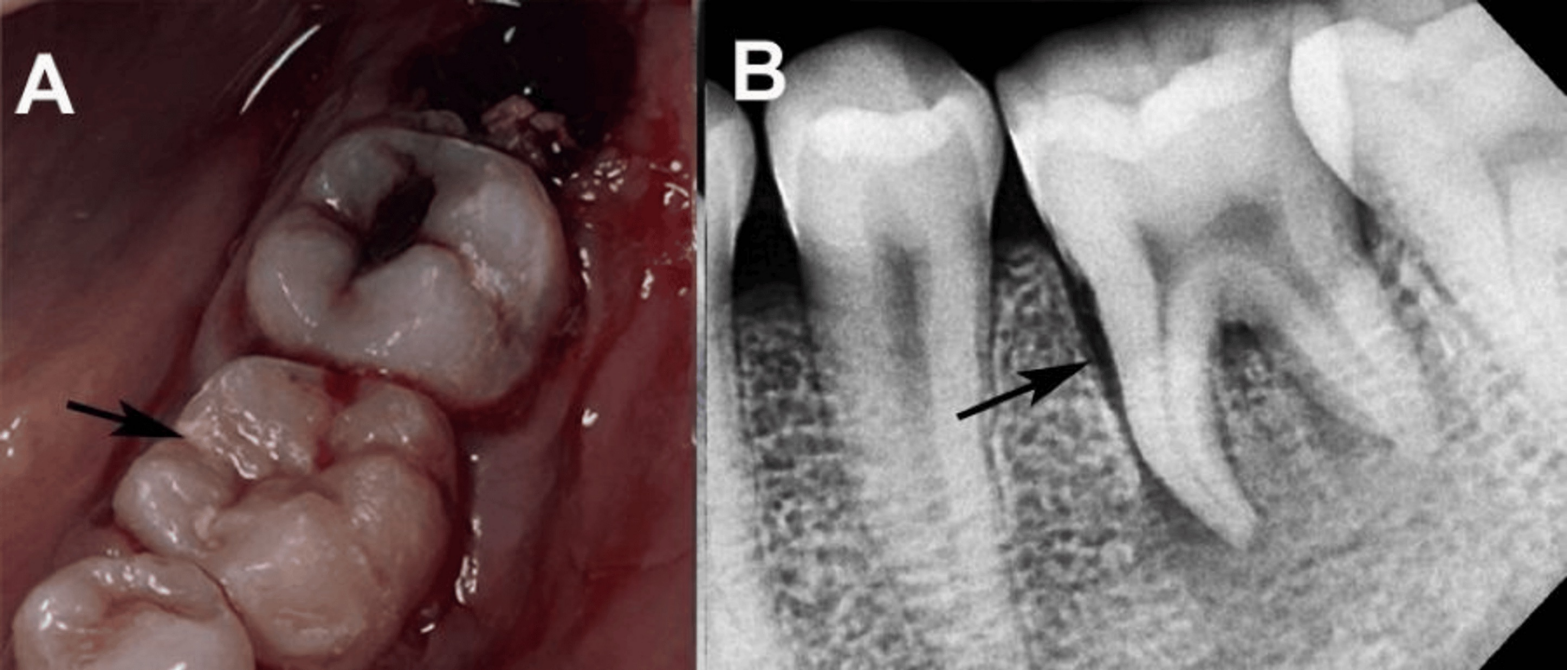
(A) Left mandibular third molar autotransplanted in the socket of left mandibular first molar (black arrow). (B) Intraoral periapical radiograph analyzing correct position of the transplanted tooth in socket (black arrow).
